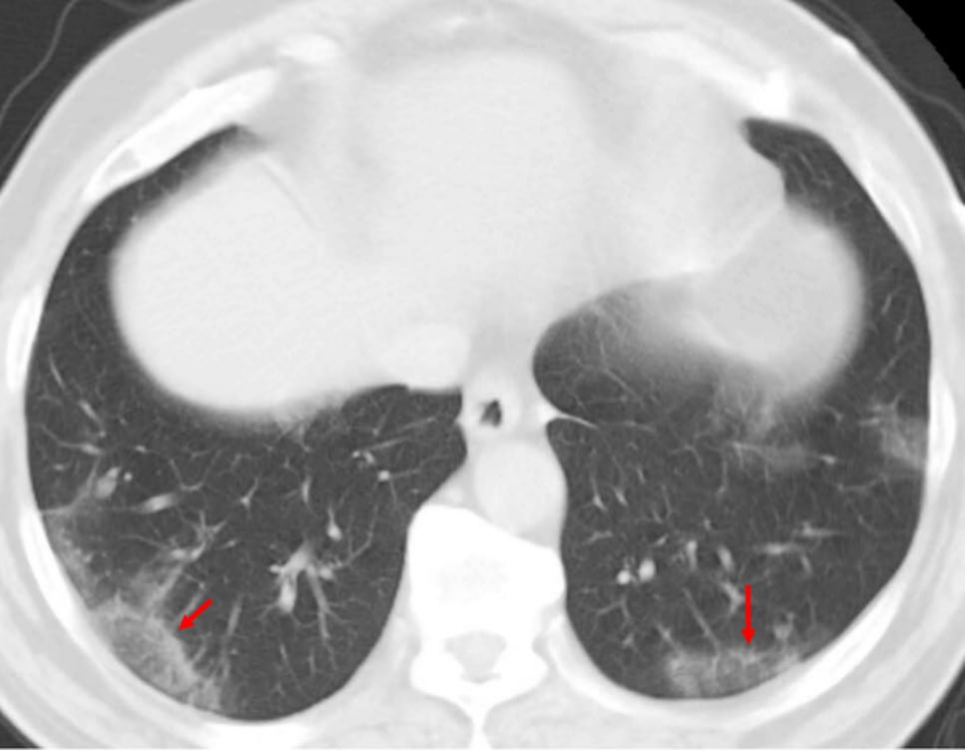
Reverse halo (atoll) sign in a patient with blunt chest trauma. Axial chest CT image demonstrates multiple subpleural pulmonary opacities with the atoll sign (arrows), likely reflecting pulmonary contusion. The patient also had non-displaced rib fractures (not shown)
